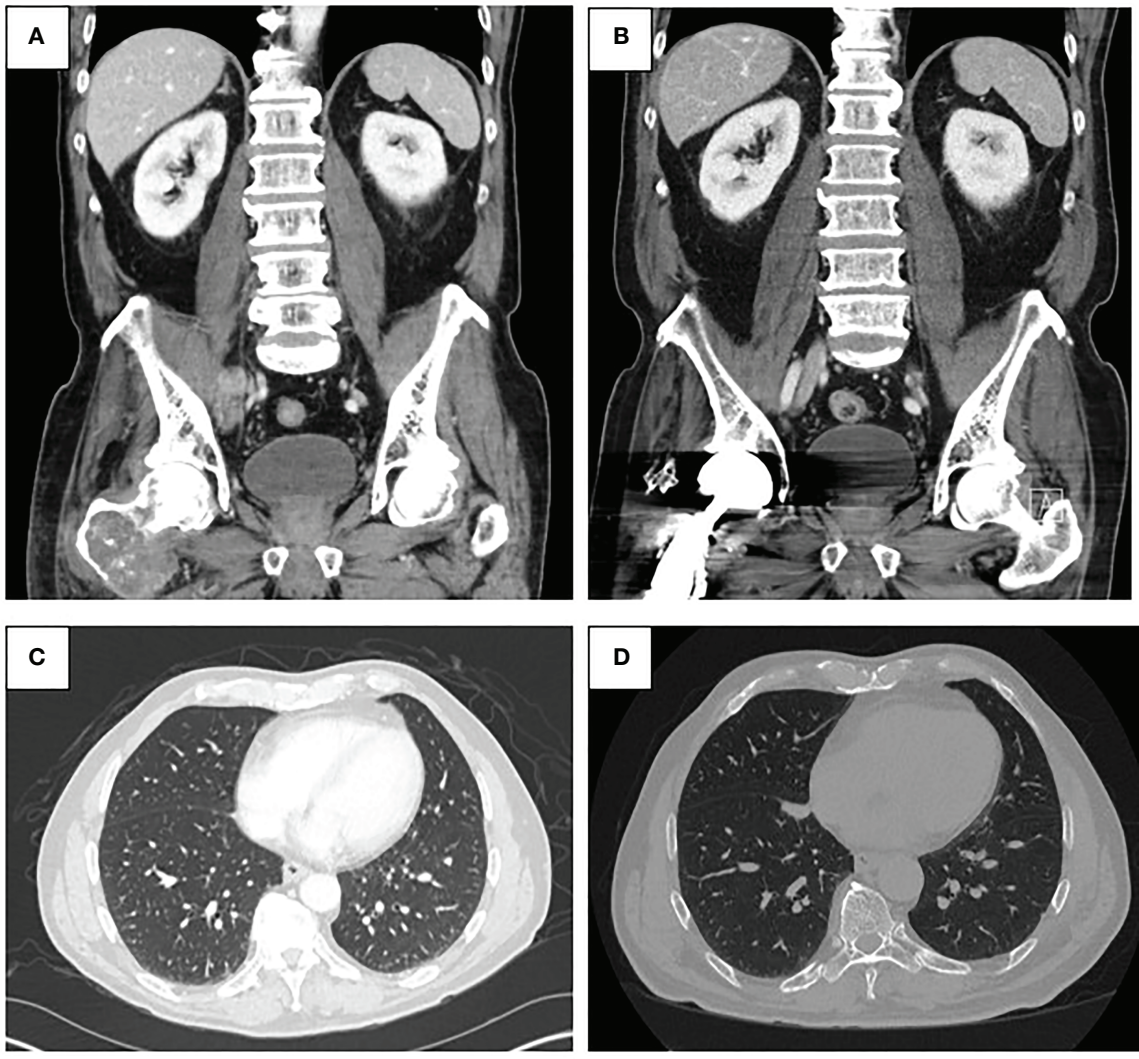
(A) Pre-treatment CT abdomen and pelvis demonstrating malignant pelvic lymph node involvement (top left). (B) Post-treatment CT abdomen and pelvis from January 2022 demonstrating resolution of pelvic lymphadenopathy (top right). (C) Pre-treatment CT chest demonstrating lung metastases (bottom left). (D) Post-treatment CT chest from January 2022 demonstrating regressed lung nodules (bottom right).
